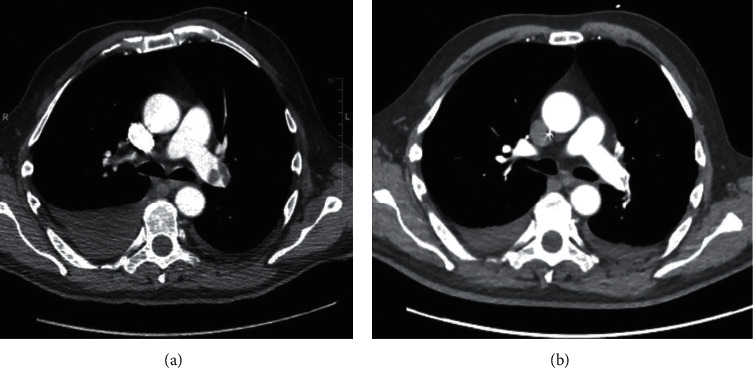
Contrast-enhanced computed tomography of the chest demonstrating a bilateral central pulmonary embolism pre-UACDT (a) and post-UACDT (b).
